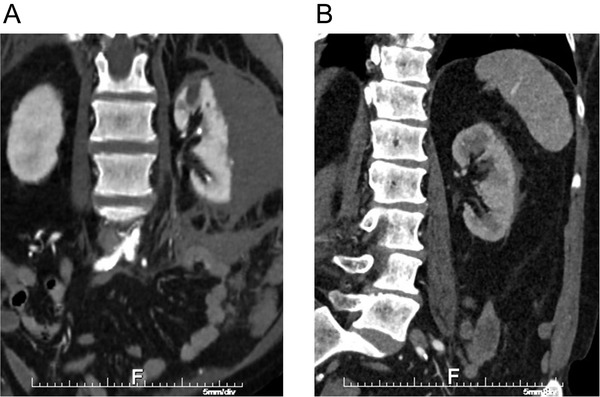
CT scan demonstrating large subcapsular and extracapsular hematomas of the left kidney post angiogram (A) and resolution of hematomas after 4 months (B)
